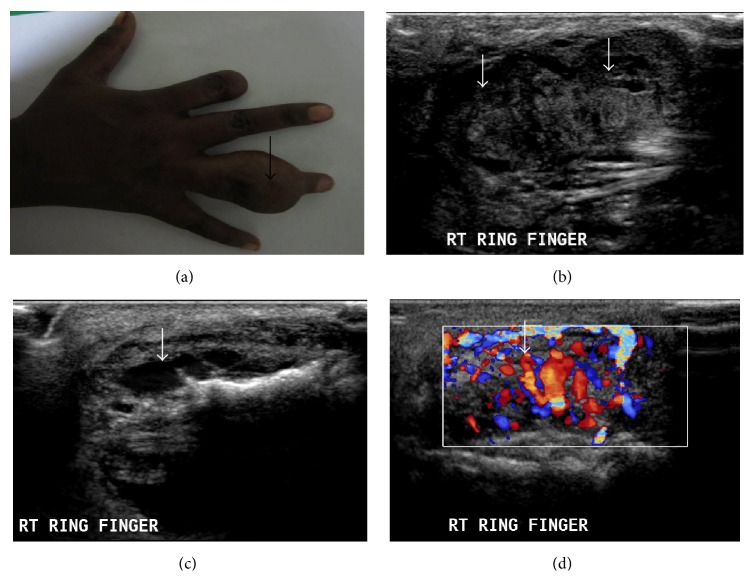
AVM. A 22-year-old female presented with compressible swelling involving middle phalanx of right ring finger ((a), arrow). Ultrasonography shows a heterogeneously hypoechoic lesion with multiple prominent linear hypoechoic channel (arrows) and irregularity of underlying bone ((b), (c)). Doppler showed markedly increased intralesional vascularity ((d), arrow).
